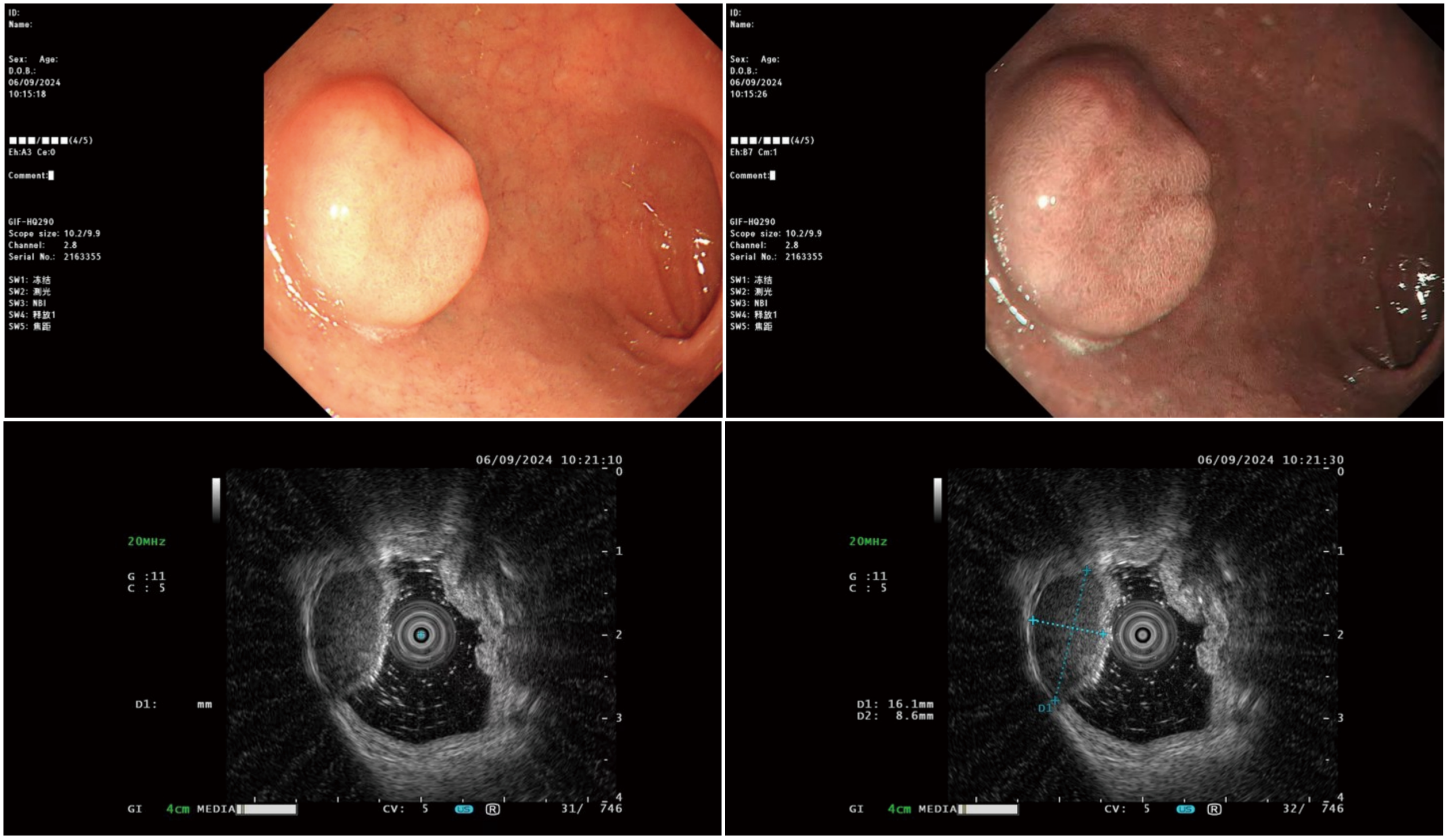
Ultrasound colonoscopy examination.
